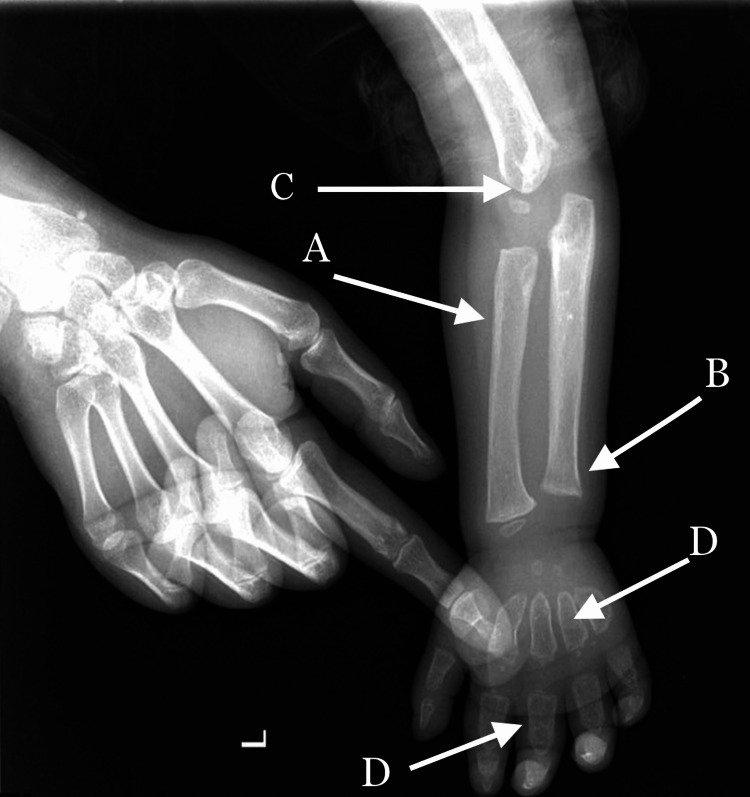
X-ray of the forearm Short diaphysis [A], flattened epiphysis [B], flamed upper limb bones [C], and bullet-shaped metacarpal bones [D]
